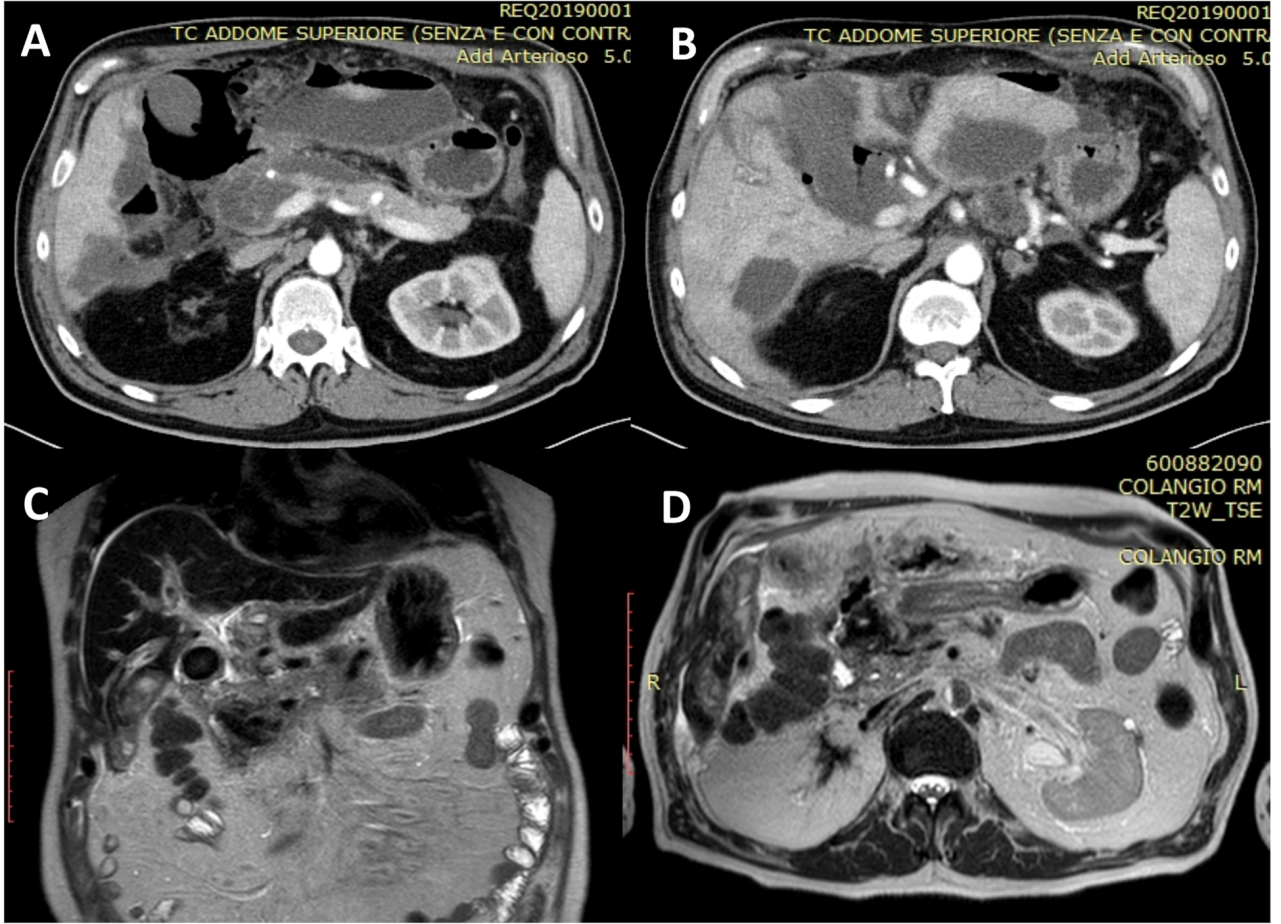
Case 3: Preoperative CT scan and MRCP. CT scan (a, b) and MRCP (c, d) showing acute gallstone cholecystitis and signs suggestive for infected pancreatic necrosis
